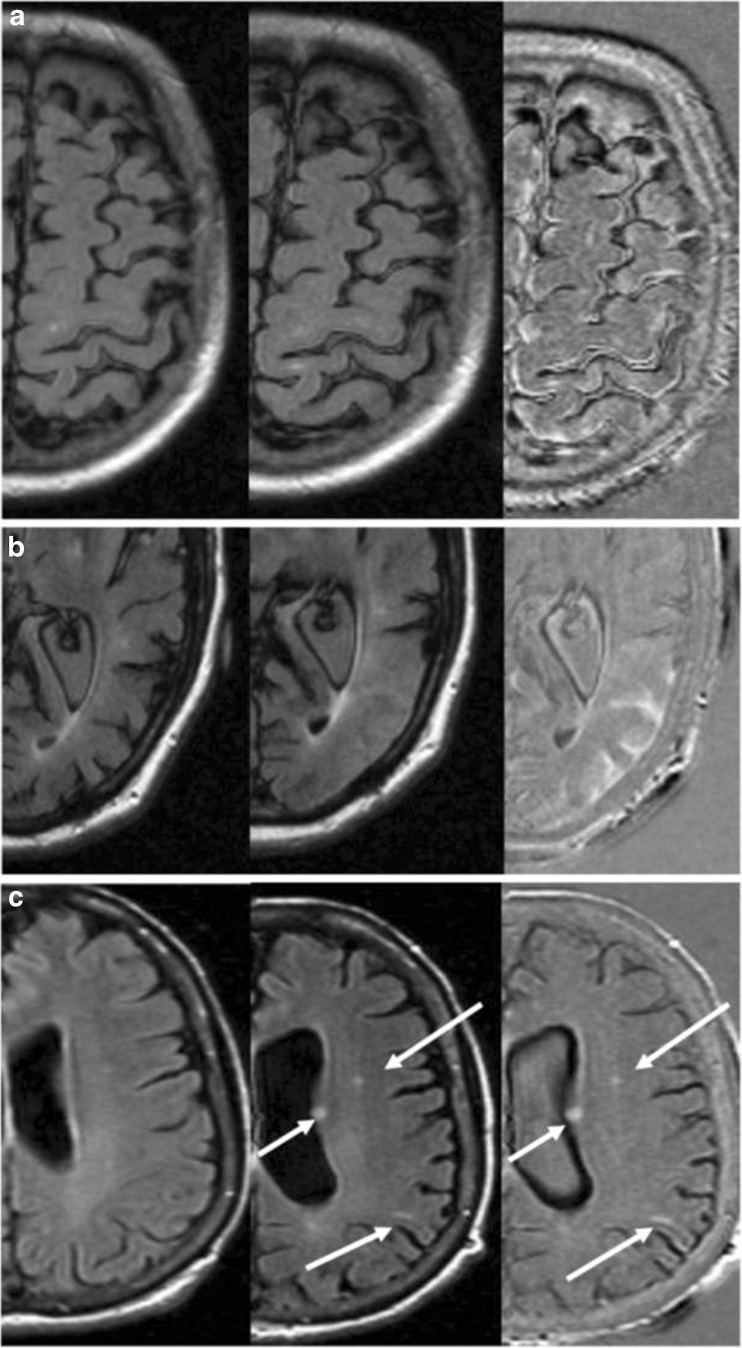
Details of three cases, with axial baseline FLAIR scan (left), the follow-up FLAIR scan (center) showing doubtful amyloid-related image abnormalities with vasogenic oedema and/or sulcal effusion (ARIA-E) findings and subtraction images (right) aiding in their detection (a), evaluation of their extent (b), and differential diagnosis (c). (a) Image artifacts prevent ARIA-E detection in FLAIR but the abnormalities are more visible on subtraction images. (b) The extension of the gyral swelling in the left occipital lobe is cumbersome to evaluate on FLAIR images but definitely more clear-cut in the subtraction images. (c) Small signal hyperintensities are visible on both FLAIR and subtraction images, hence their vascular origin may be excluded
